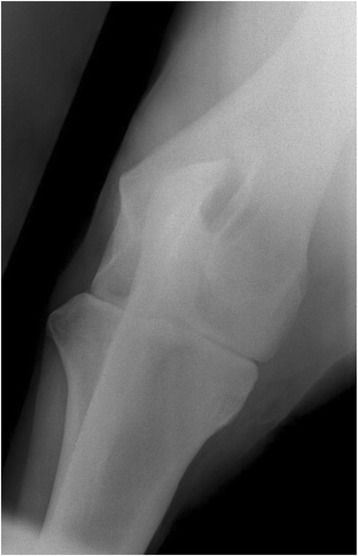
Craniocaudal radiographic views of the elbow of a 3 year old thoroughbred gelding with lateral partial avulsion of the ulnaris lateralis and enthesiopathy of the lateral epicondyle of the humerus. No radiographic abnormalities were identified
